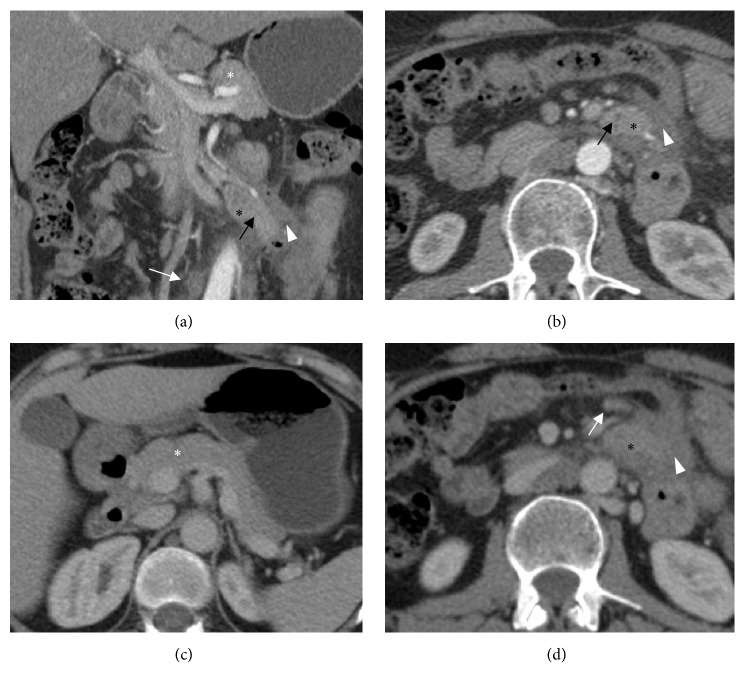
CT scan performed six weeks after admission. (a-b) Coronal and axial image in the arterial phase. (c-d) Axial images in the portal venous phase. Intra-abdominal free fluid is completely resolved. Heterotopic pancreas* (black asterisks)* in the mesentery with attenuation characteristics after intravenous contrast comparable to the orthotopic pancreas* (white asterisks)*. Moreover, presence of a central duct is suggested* (black arrow)*. There is an apparent decrease in inflammatory changes* (white arrowheads)* surrounding the heterotopic pancreas, though several adjacent mesenteric lymph nodes have increased in size* (white arrows)*.
